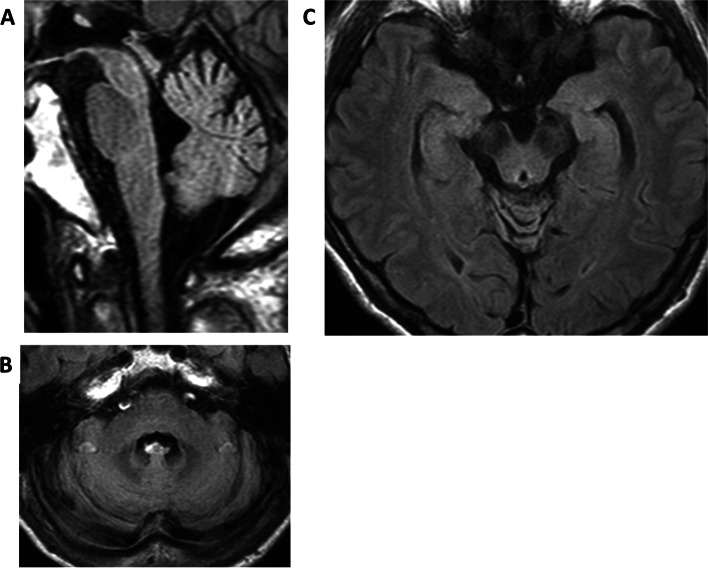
Brain magnetic resonance fluid-attenuated inversion recovery (MRI-FLAIR) images. The axial and sagittal views reveal mild atrophy of the cerebellar vermis and hemisphere, but no atrophy of the brain stem. A, B Atrophy of the cerebral cortex or hippocampus is not observed. (C)
